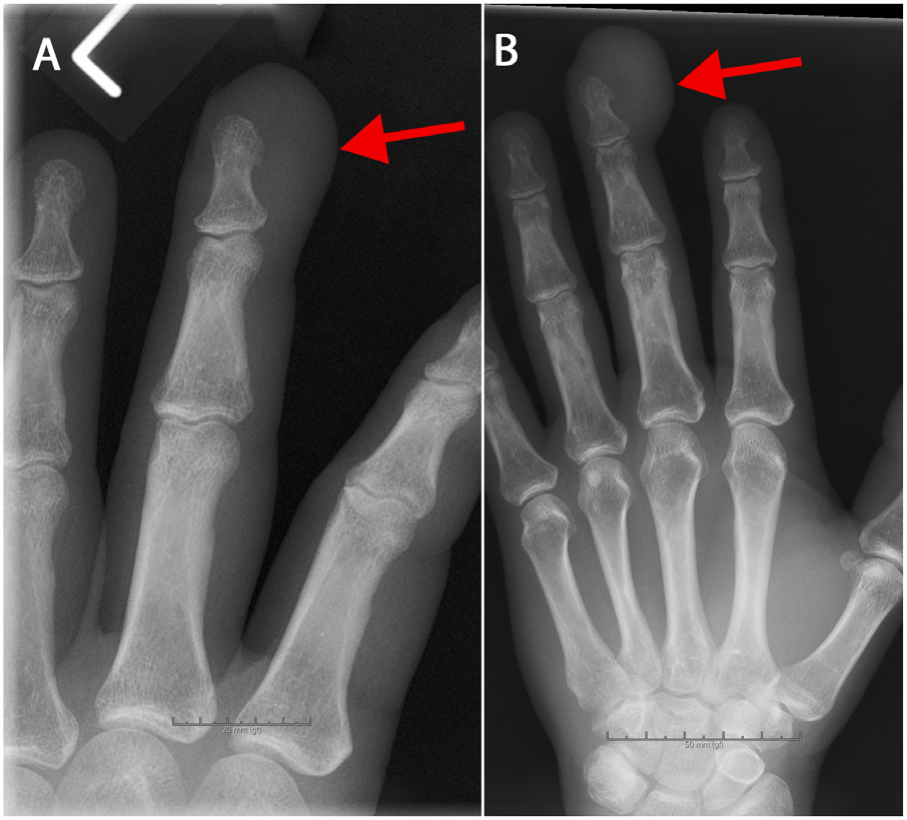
(A) Plain radiograph of the left third finger (2012) shows soft tissue swelling at the fingertip, without evidence of bone erosion or a visible foreign body (red arrow). (B) Follow-up radiograph of the same finger in 2015 demonstrates progression of soft tissue swelling compared with the 2012 image, along with subtle bone involvement, still without detectable foreign body (red arrow).
